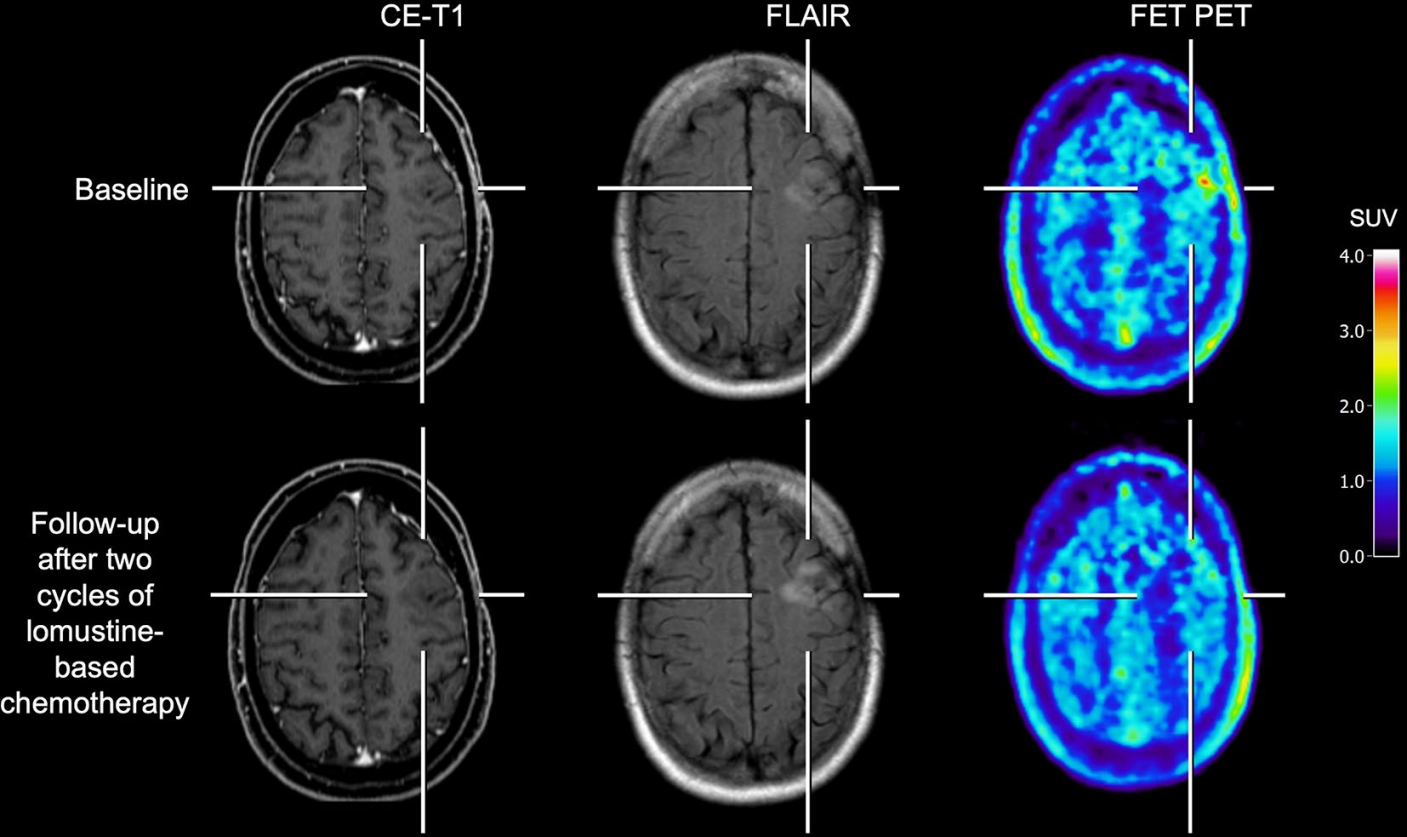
Contrast-enhanced MRI and FET PET of a 52-year-old male patient with a left frontal IDH-mutant, 1p/19q-codeleted oligodendroglioma of the WHO CNS grade 2 after resection and radiotherapy (top row). The corresponding FET PET scan shows residual metabolic activity (maximum tumor-to-brain ratio, 2.1). After two cycles of adjuvant chemotherapy with procarbazine and lomustine, the follow-up MRI (bottom row) shows an increasing FLAIR signal alteration without contrast enhancement, suggesting tumor progression. In contrast, the follow-up FET PET shows no increased metabolic activity, indicating a metabolic response. During adjuvant chemotherapy, the hyperintense FLAIR signal regressed partially, and the patient was free of tumor progression for more than two years
